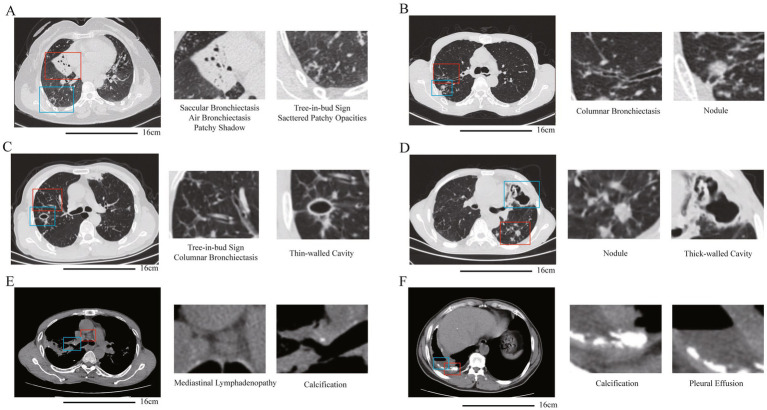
Comparative chest CT imaging features of mycobacterial lung diseases. (A) (Axial): Diffuse airway changes in NTM-LD: Red: Cystic bronchiectasis (thin-walled clusters), consolidation/air bronchograms; Blue: Tree-in-bud sign, patchy opacities. (B) (Coronal): Bronchial dilation and nodules: Red: Cylindrical bronchiectasis (“signet-ring” sign); Blue: Peripheral nodules (2–3 cm). (C) (Sagittal): Airway/cavitary lesions: Red: Tree-in-bud sign + cylindrical bronchiectasis; Blue: Thin-walled cavity (smooth margins). (D) (Axial): Nodular/cavitary pathology: Red: Large nodules (≥3 cm, irregular); Blue: Thick-walled cavity + consolidation. (E) (Coronal): Mediastinal/calcified lesions: Red: Mediastinal lymphadenopathy; Blue: Punctate calcifications (bronchovascular). (F) (Axial): Pleural/calcification features: Red: “Popcorn” calcification (parenchymal); Blue: Pleural effusion + pneumothorax.
